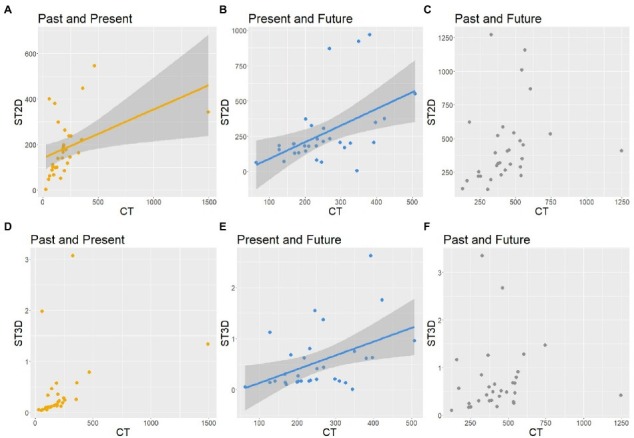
Distance between the past and present time zones in ST as a function of the distance in CT. Each dot expresses the past, present, and future positions of a participant. The gray areas surrounding the regression lines indicate the confidence interval. (A) Past-Present distance on the screen in ST. (B) Present-Future distance on the screen in ST. (C) Past-Future distance on the screen in ST. (D) Past-Present distance in 3D space in ST. (E) Present-Future distance in 3D space in ST. (F) Past-Future distance in 3D space in ST.
